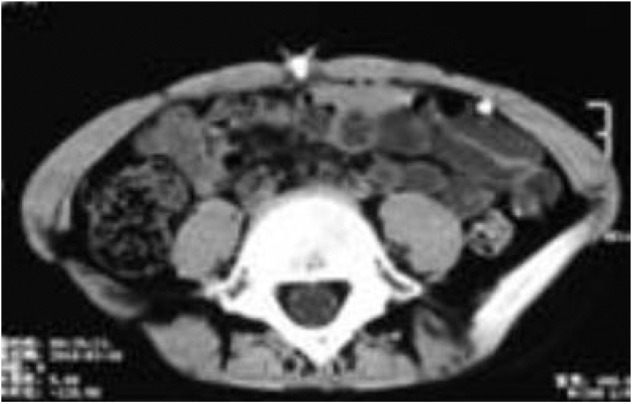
Abdominal CT revealing that the effusion disappeared.
